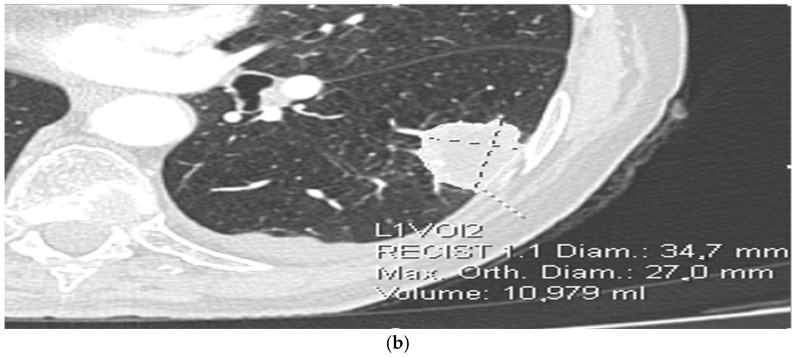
(a) Extraction of radiomic features from CT images. (b) Extraction of radiomic features from CT images.
